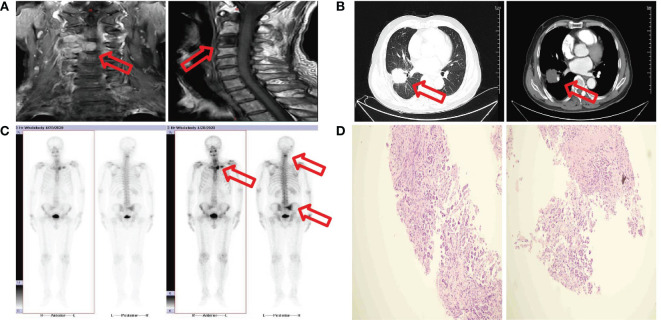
(A) MRI image shows C3/C4 vertebral bodies and their right attachments presented bone destruction with soft tissue mass. (B) CT image shows a mass measure 3.5 cm×3.9 cm in the right lower lobe. (C) Bone scan image shows multiple bone metastases. (D) Fine needle aspiration biopsy show adenocarcinoma.
